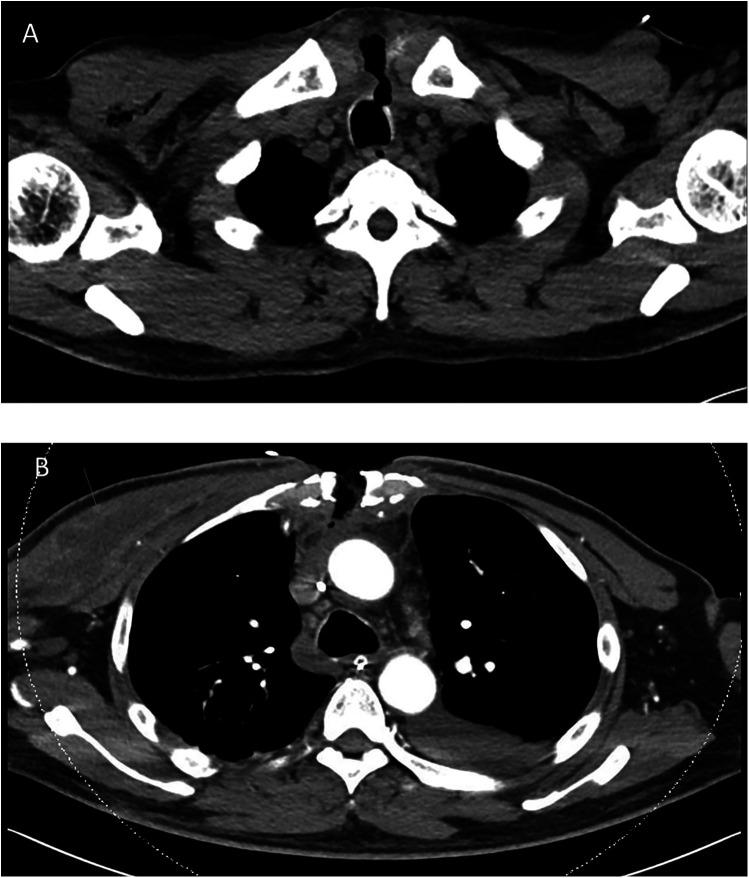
Patient's chest CT. (A) chest CT performed during his second readmission showing a tracheocutaneous fistula from the upper trachea to the subcutaneous sternum along the sternotomy defect. There was subcutaneous emphysema along the manubrium sternum, posterior sternum, and mediastinum, extending over the right chest wall. No large mediastinal collection was noted in the study. (B) Follow-up chest CT performed 1 week later showing interval development of a pectoralis major abscess (arrow), which required further surgical drainage.
